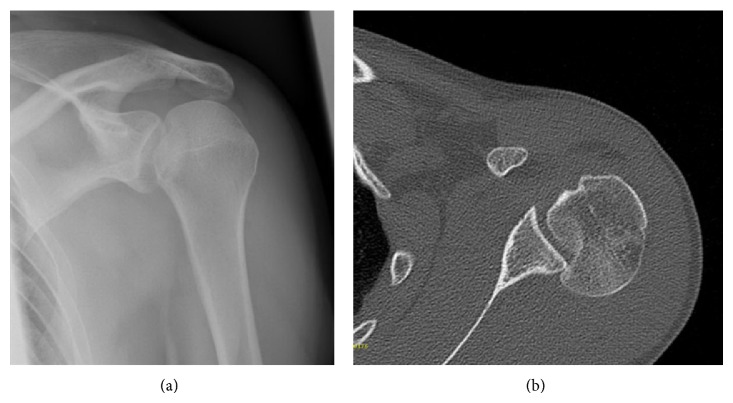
X-rays (a) and CT (b) showing the joint fixed in dorsal dislocation with the presence of a reversed Hill-Sachs defect.
